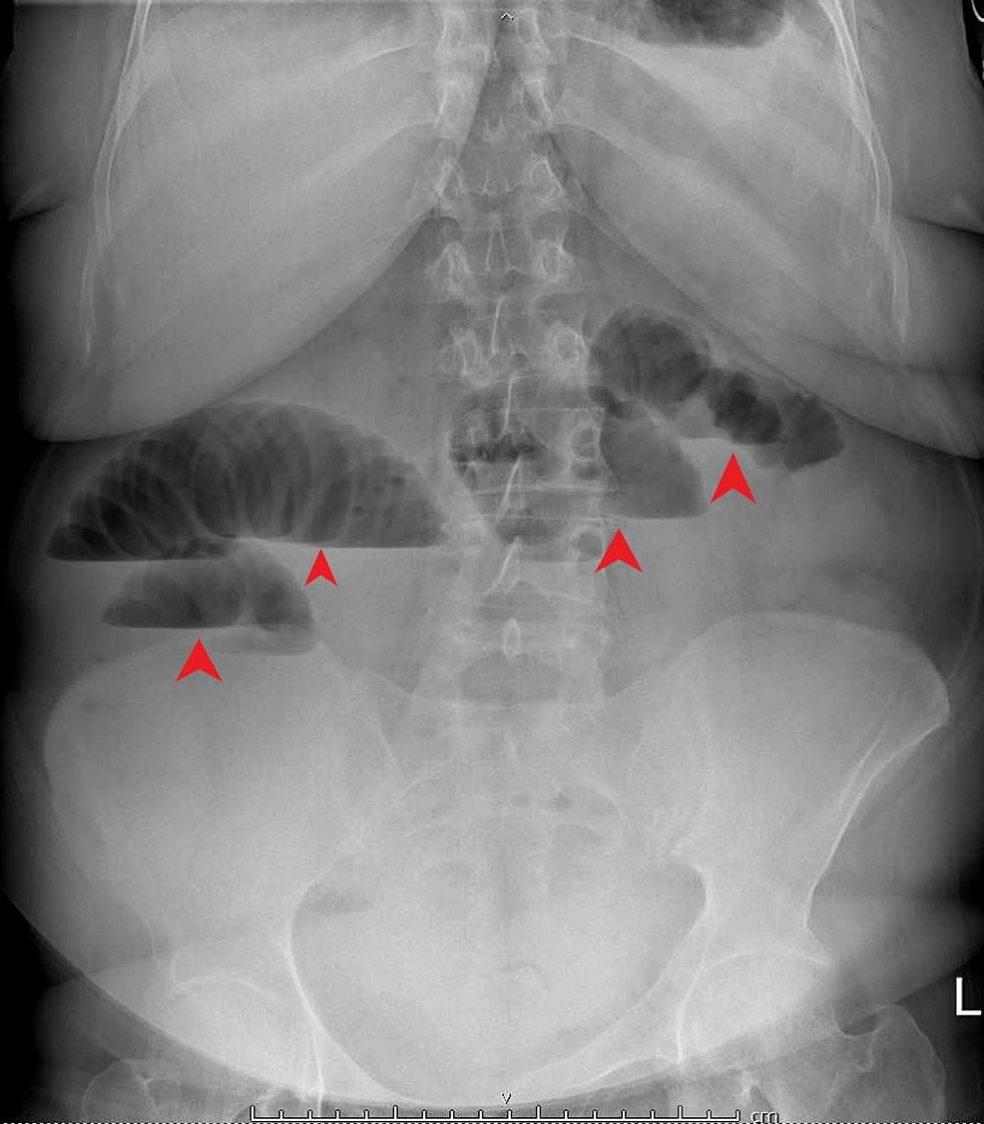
Abdominal X-ray (red arrowheads) demonstrate dilated bowel loops (likely small bowel) with multiple fluid level noted suggesting small bowel obstruction
